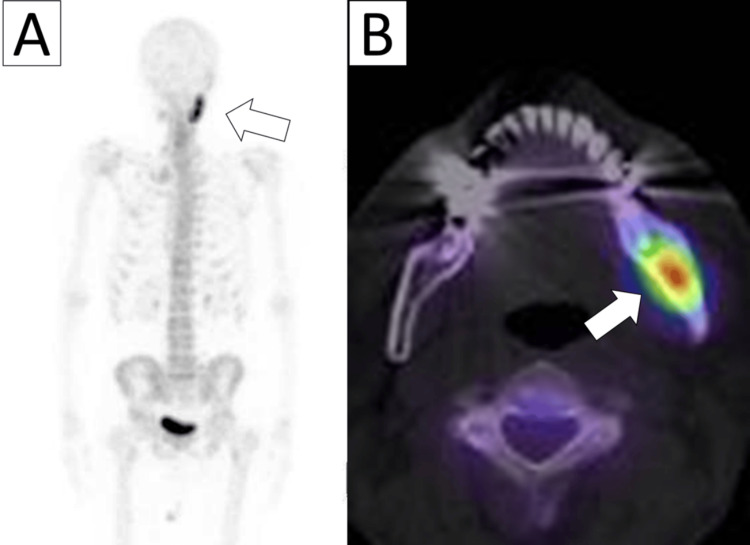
Tc-99m scintigraphy at the first visit to our department A: Increased tracer uptake is observed in the left mandible (arrow), with no abnormal findings in the rest of the skeletal system B: SPECT showing intense tracer accumulation in the left mandible (arrow) SPECT: Single Photon Emission CT
